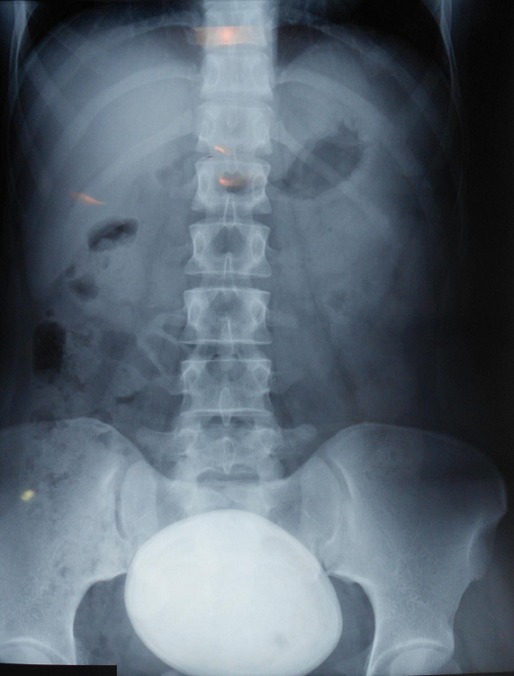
X-ray of urinary tract, showing a calcic opacity in the entire bladder
